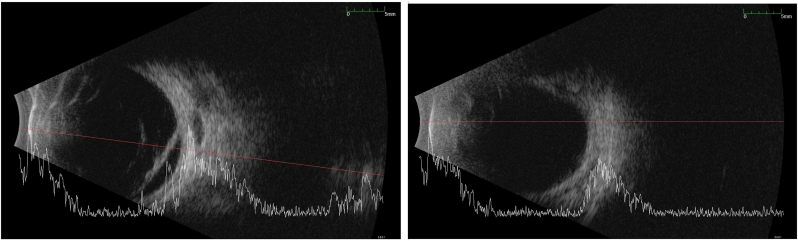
Left Panel: Pre-operative ultrasound image demonstrates retinal detachment. Right Panel: Ultrasound image following scleral buckle surgery of the same eye shows slightly elongated eye with attached retina.
